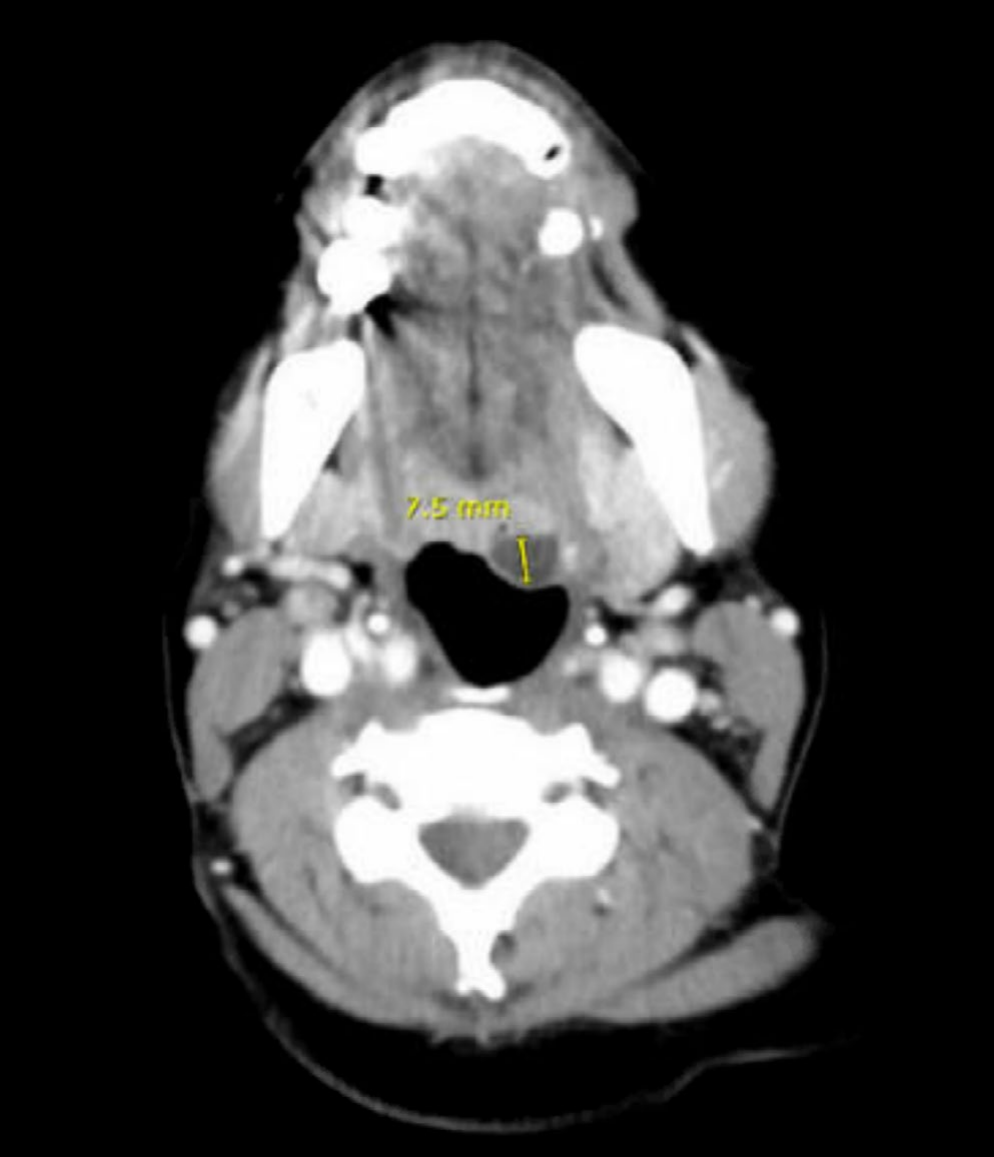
CT NECK‐soft tissues showing a 0.75 cm non‐obstructive vallecular cyst.
